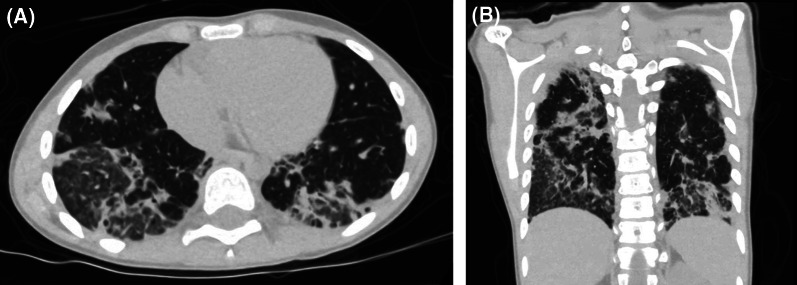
CT thorax demonstrates predominantly peripheral bilateral infiltrate with combined interstitial reticular, nodular, and ground-glass opacities alongside the confluent regions of the lower lobes. A Transverse plane; B coronal plane
